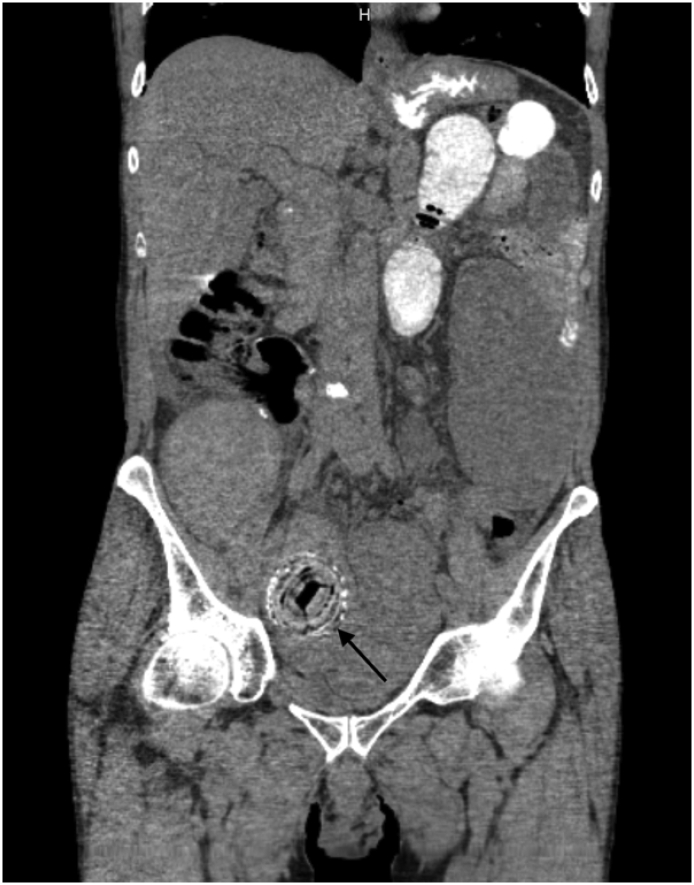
Coronal CT scan with oral contrast - dilated small bowel loops, foreign body (arrow).
